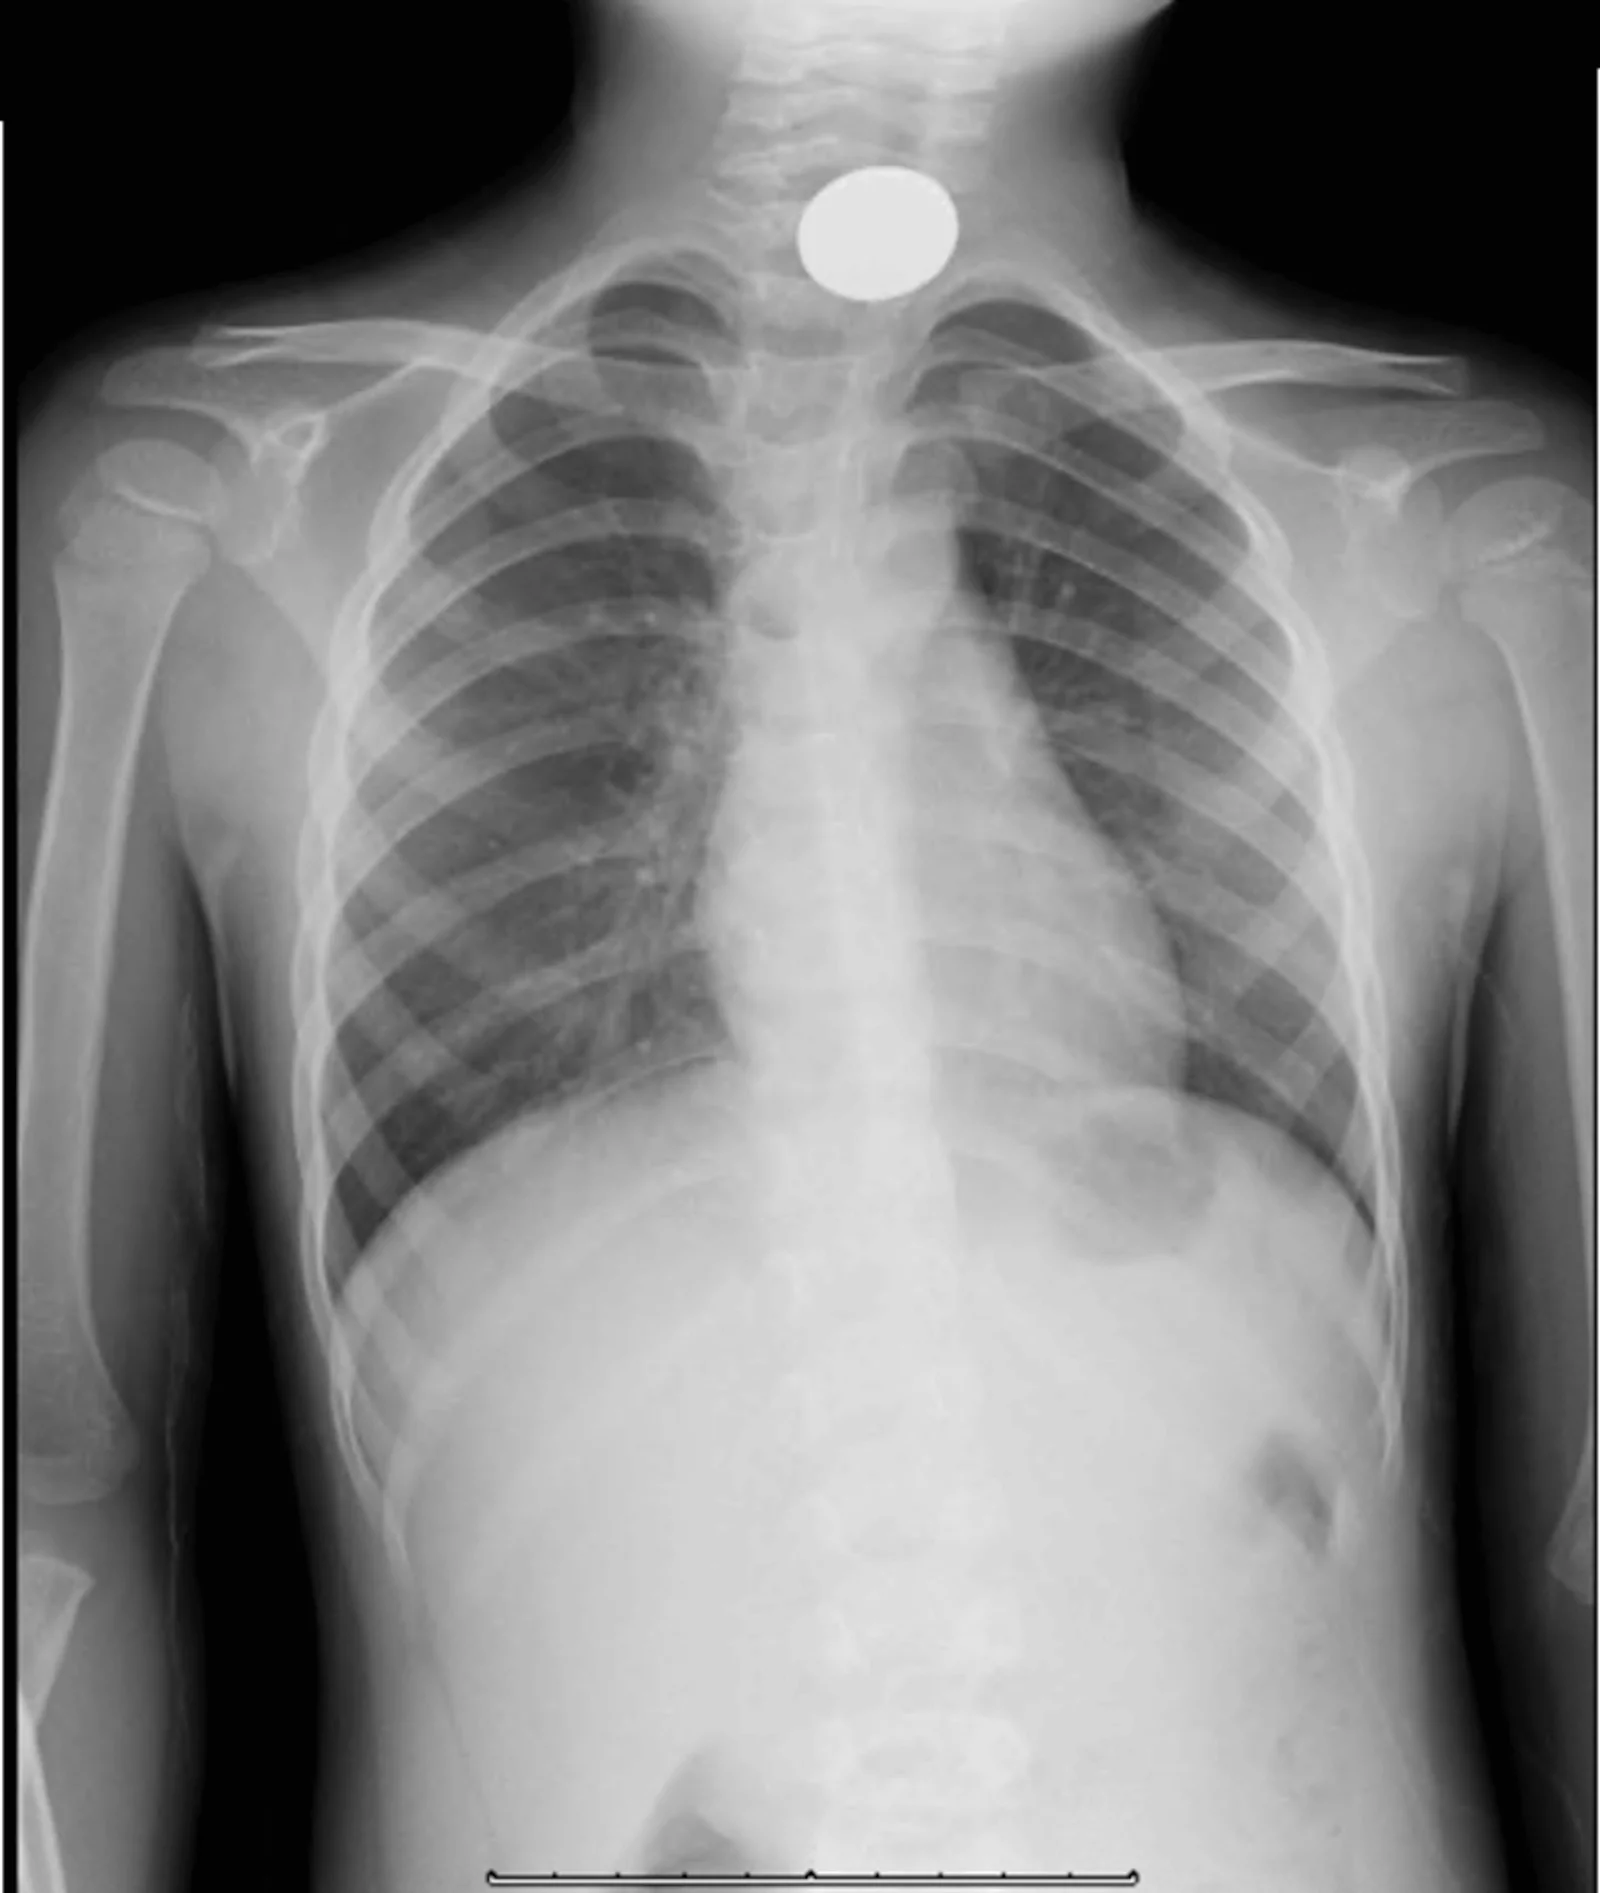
Anteroposterior chest radiograph showing a round coin impacted at the level of the sixth cervical vertebra (C6), corresponding to the cricopharyngeus
